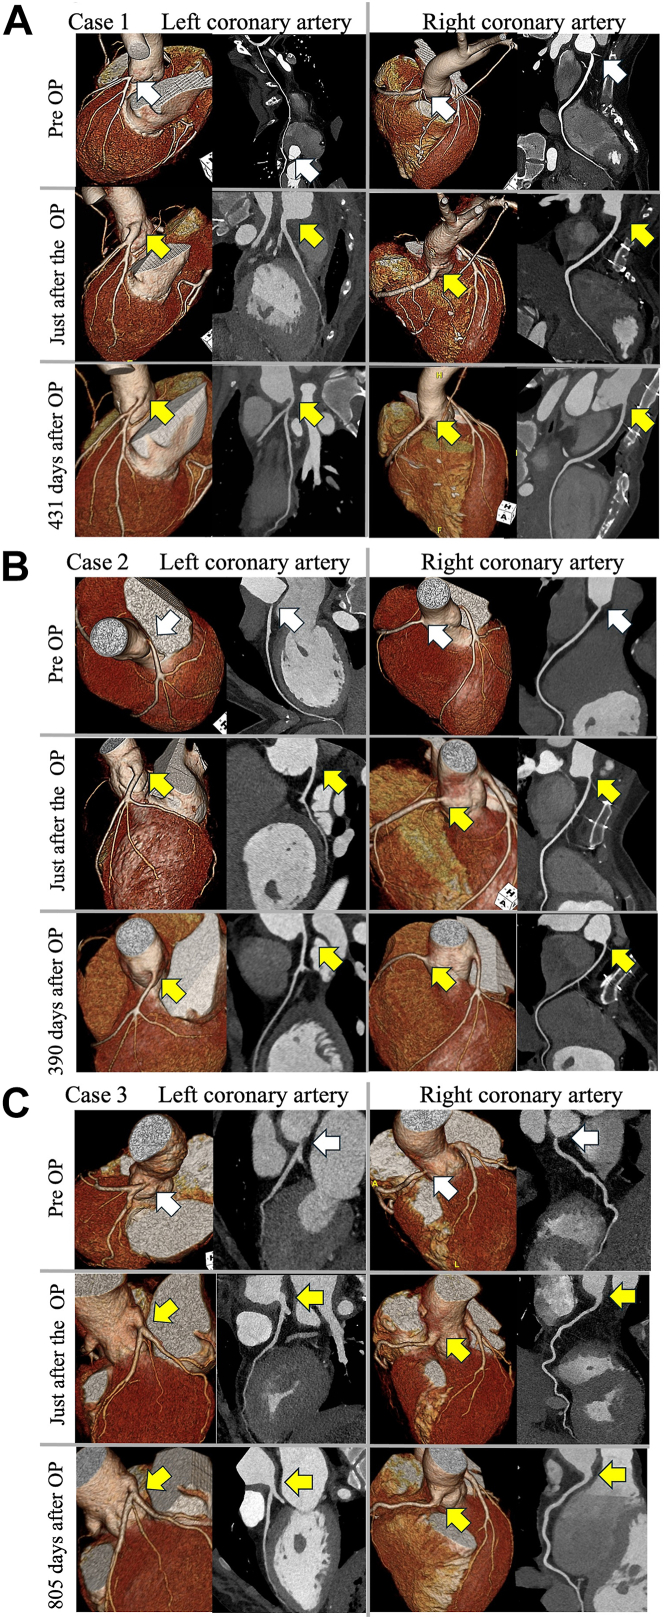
Preoperative and postoperative multiplanar reconstruction computed tomography images of each coronary artery. (A) Patient 1. (B) Patient 2. (C) Patient 3. White arrows indicate coronary ostial lesions. Yellow arrows indicate enlargement of the left main coronary artery ostium by an external iliac artery patch. (OP, operation; Pre OP, preoperative.)
